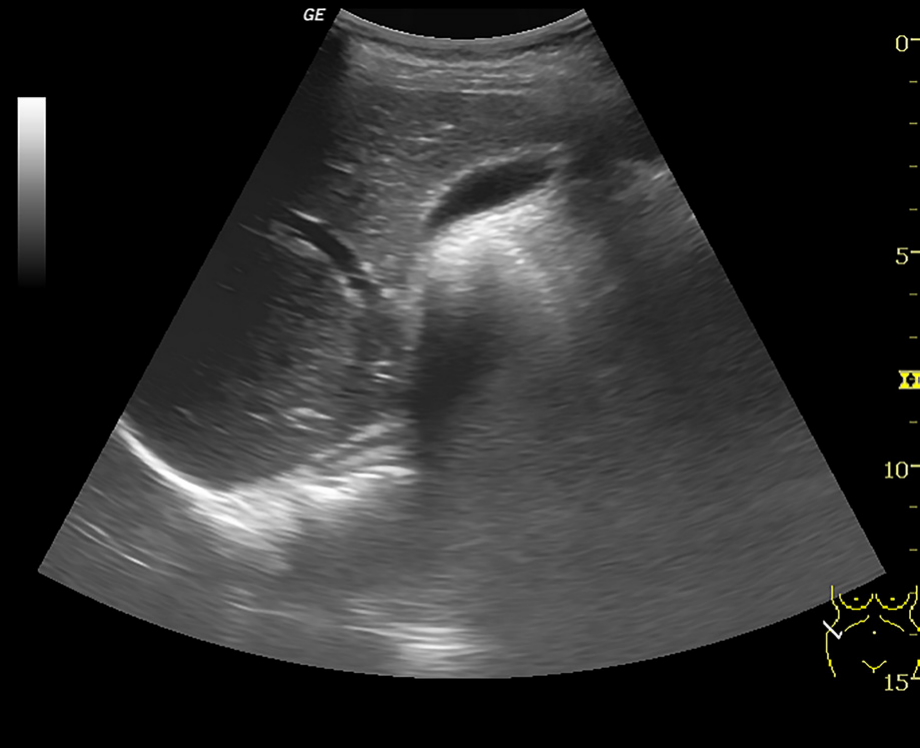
Transverse ultrasound of right hepatorenal space showing
